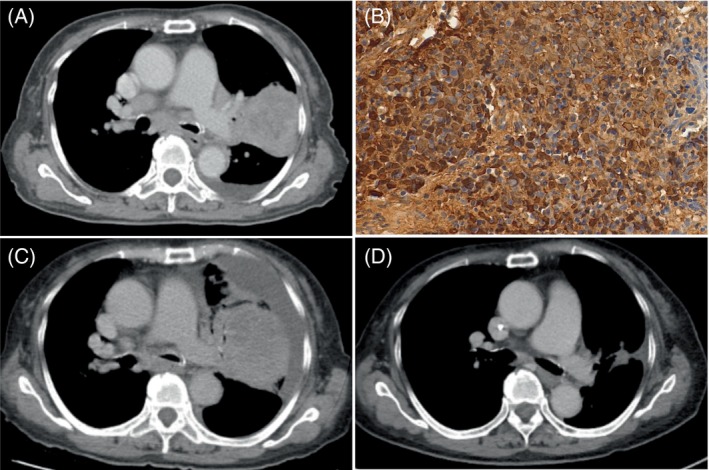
(A) Chest CT showing an 11.4 cm mass in the left upper lung, indicative of stage IVA disease. (B) Epstein–Barr encoded region in situ hybridization, highlighting positive tumor cell nuclei (200× magnification). (C) Progression of the disease after 8 months and 12 cycles of treatment, evidenced by increased mass size and pleural effusion. (D) Marked reduction in tumor size and pleural effusion following four cycles of gemcitabine, carboplatin, and pembrolizumab chemotherapy. CT, computed tomography.
